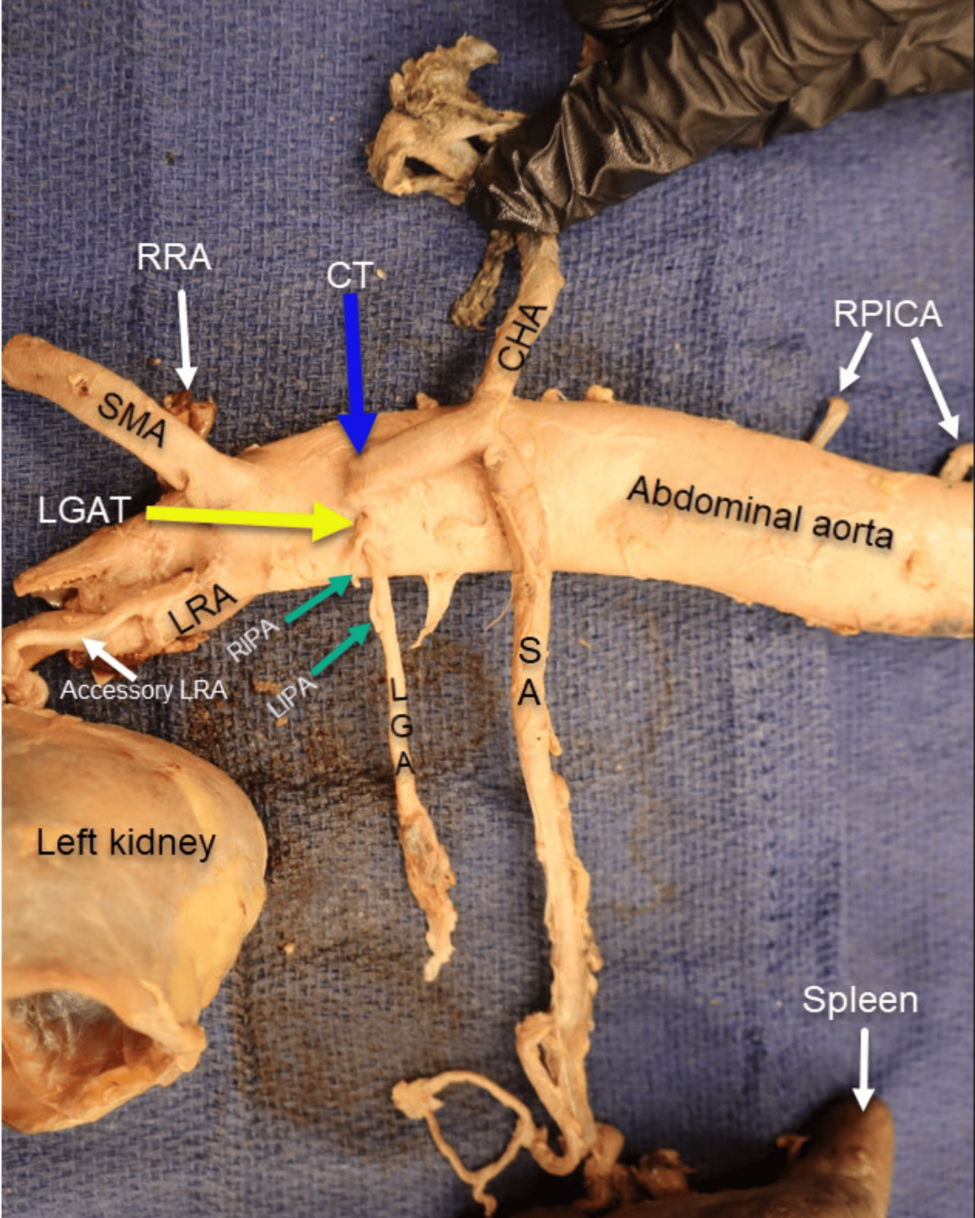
Aberrant left gastric artery trunk. The left gastric artery (LGA, yellow arrow) arises directly from the abdominal aorta (AA) as a distinct trunk. Both the right and left inferior phrenic arteries (RIPA and LIPA, green arrows) originate from this anomalous LGA trunk. The celiac trunk (CT, blue arrow) gives rise to two primary branches: the splenic artery (SA) and the common hepatic artery (CHA). Inferior to the CT, the superior mesenteric artery (SMA) emerges from the AA. The right renal artery (RRA) and left renal artery (LRA) also arise from the AA, with an accessory left renal artery observed branching from the LRA. Additionally, the right posterior intercostal arteries (RPICA) are seen originating from the thoracic aorta.
